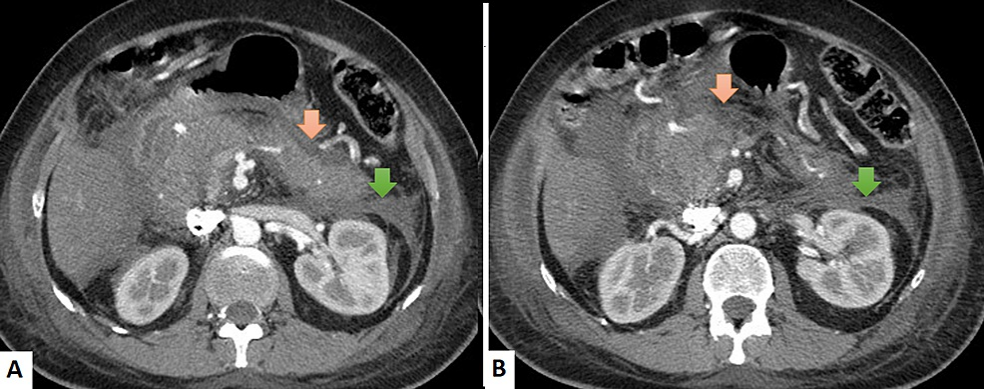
Contrast-enhanced CT images in axial plans showing acute pancreatitis peripancreatic fat standing (orange arrow), fluid collection associated with perinephric fascial thickening (green arrow) evocative of severe pancreatitis (A and B)
